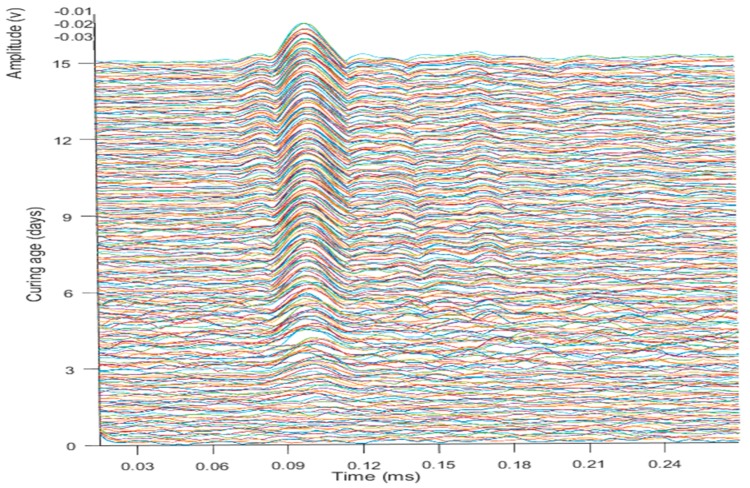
Amplitude rise pattern with curing time for ultrasonic waves data.
